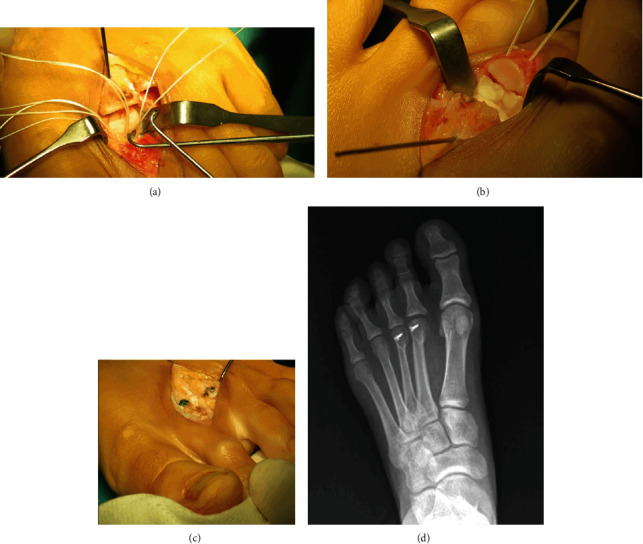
Surgical view: (a) plantar plate sutures; (b) osteosutures of the bone fragments; (c) approach on the second and third metatarsal bone; (d) postoperative X-ray at 2-year follow-up.
